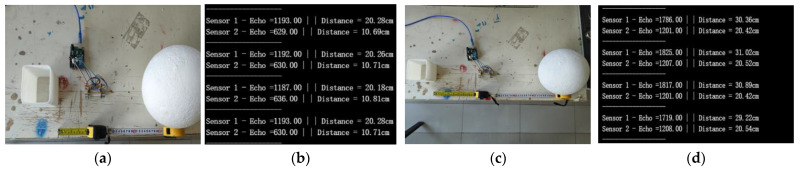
The actual distance measured by ultrasonic ranging. (a) Group A experiments; (b) Group A experiment results read via the Arduino IDE; (c) Group B experiments; (d) Group B experiment results read via the Arduino IDE.
